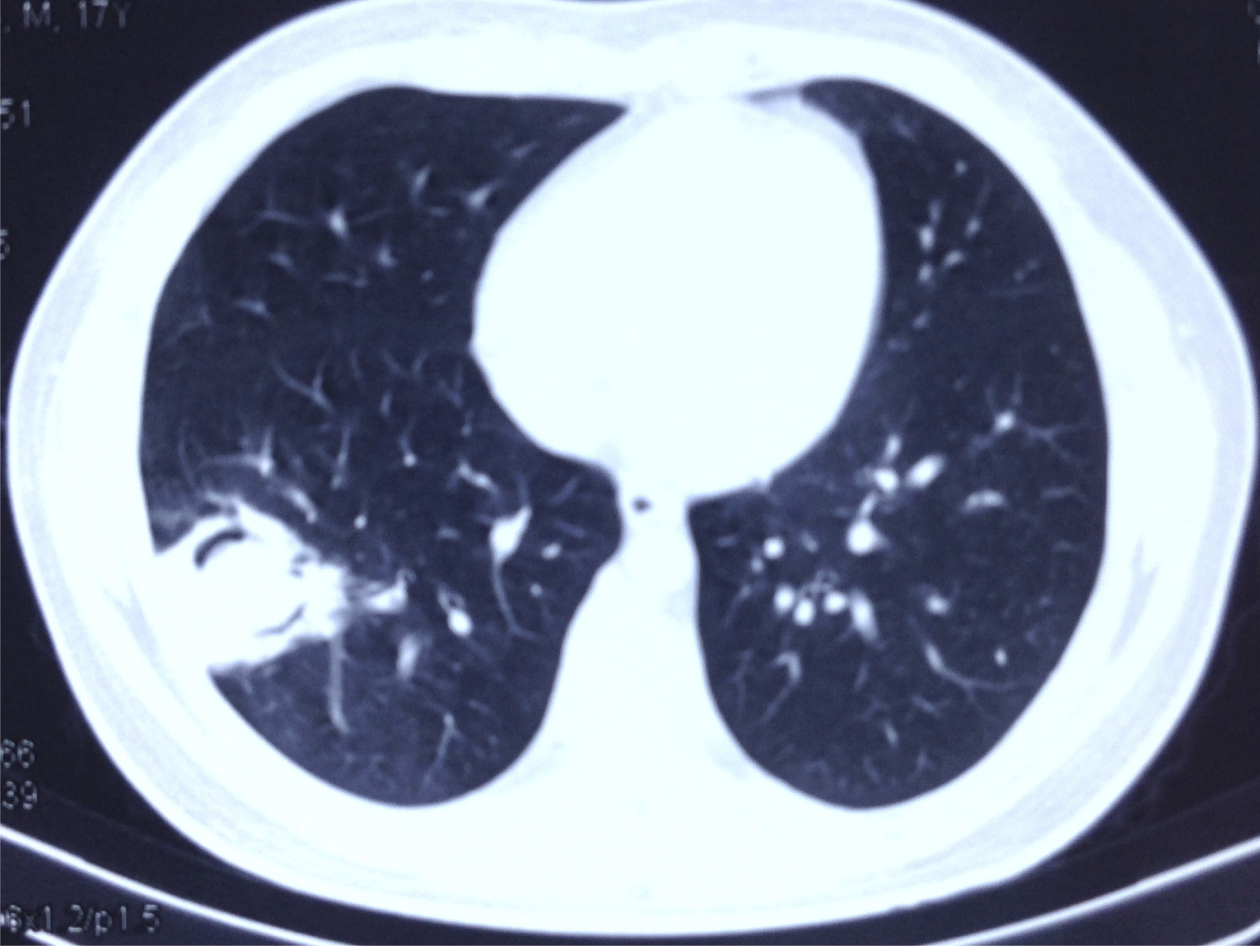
Chest CT of a 16-year-old male survival patient. On day 40 after disease onset, chest CT showed a typical crescent sign and halo sign indicative of invasive pulmonary aspergillosis. The patient recovered from invasive pulmonary aspergillosis after voriconazole treatment for 12 months.
